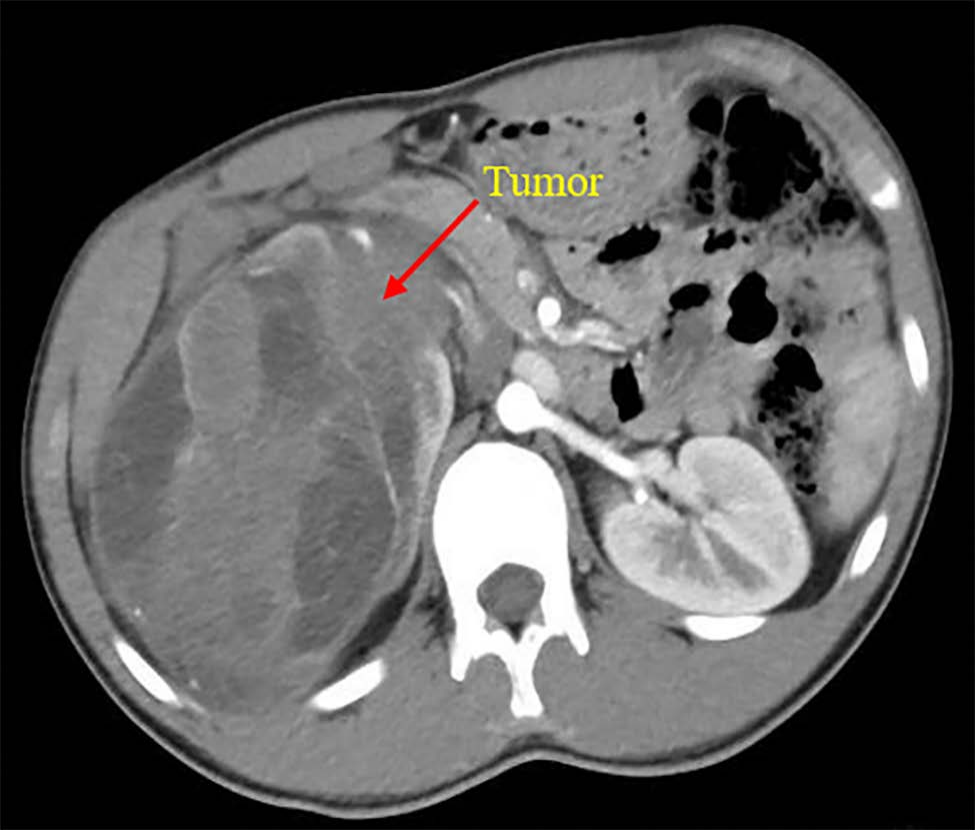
The first preoperative MRI of the patient shows a large tumor of the right kidney. MRI = magnetic resonance imaging.
